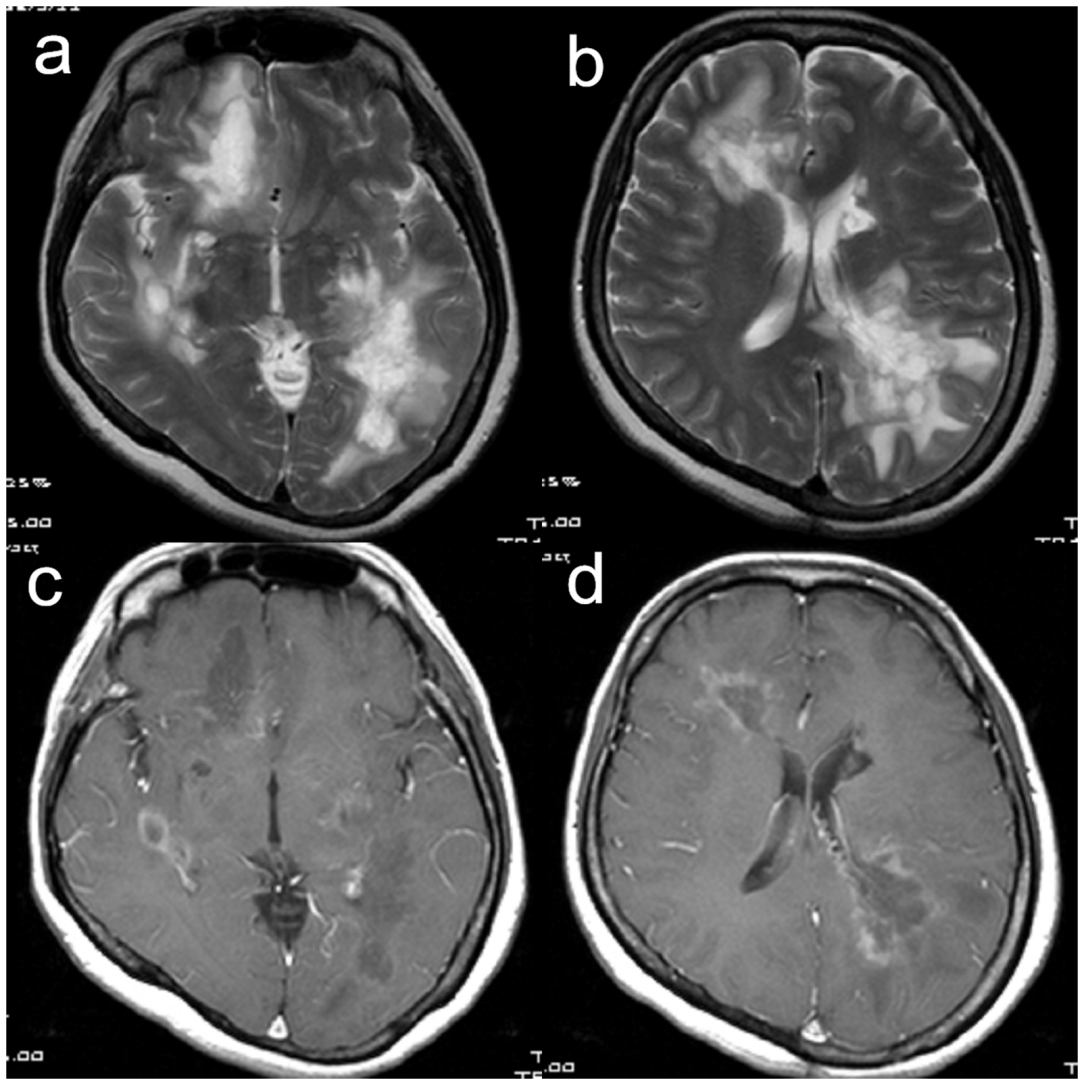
T2-weighted magnetic resonance images (a, b) of the patient #4. They show multiple large hyperintensity lesions over periventricular and juxtacortical area of right frontal and left parietal lobes with perifocal edema. Thalamus, basal ganglion and internal capsule are also involved. Gadolinium-enhanced T1-weighted (c, d) images of the patient also show ring and heterogenous enhancement in right frontal and left parietal lobes.
